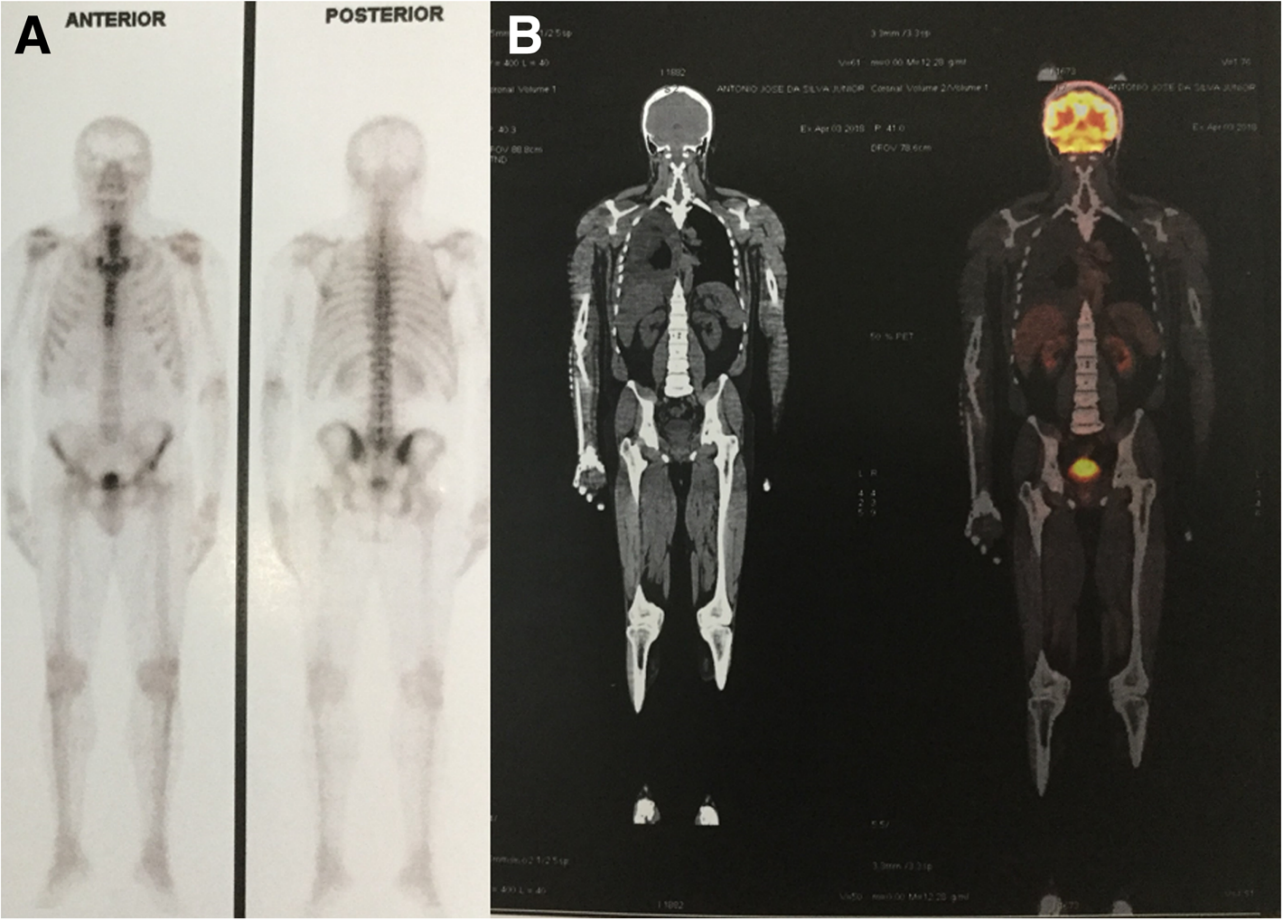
a Bone scintigraphy with heterogeneous distribution of the radiopharmaceutical M-methylenediphosphonate technetium-99m throughout the skeleton, with hyperconcentration of the tracer in the shoulders suggesting degenerative changes. b Positron emission tomography-computed tomography with fluorodeoxyglucose demonstrating multiple lytic bone lesions without a sclerosis halo or cortical bone lysis, some of them showing discrete glycolytic hypermetabolism (maximum standardized uptake value, 2.9)
